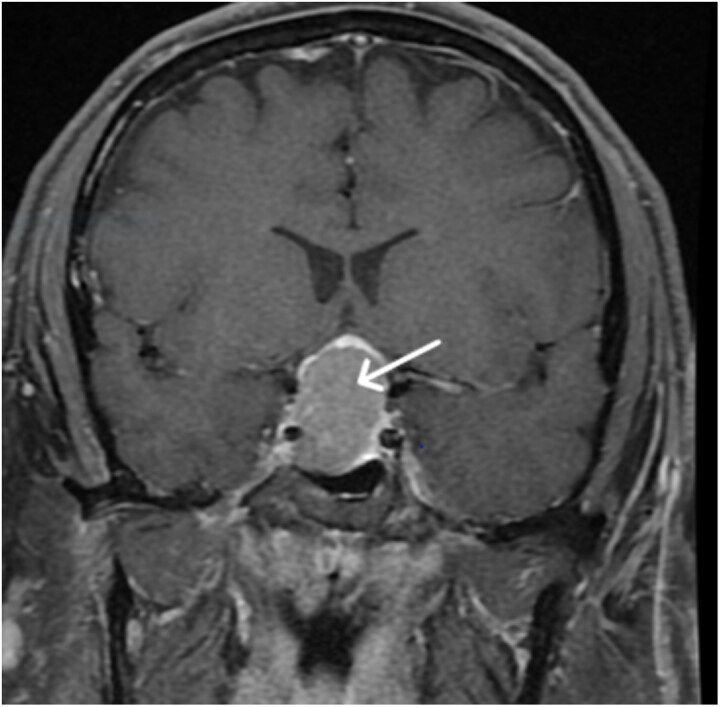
Magnetic resonance imaging of the brain before treatment with cabergoline for patient 2. Here, the pituitary macroadenoma is visualized bulging into the right cavernous sinus and resulting in mass effect on the optic chiasm.
